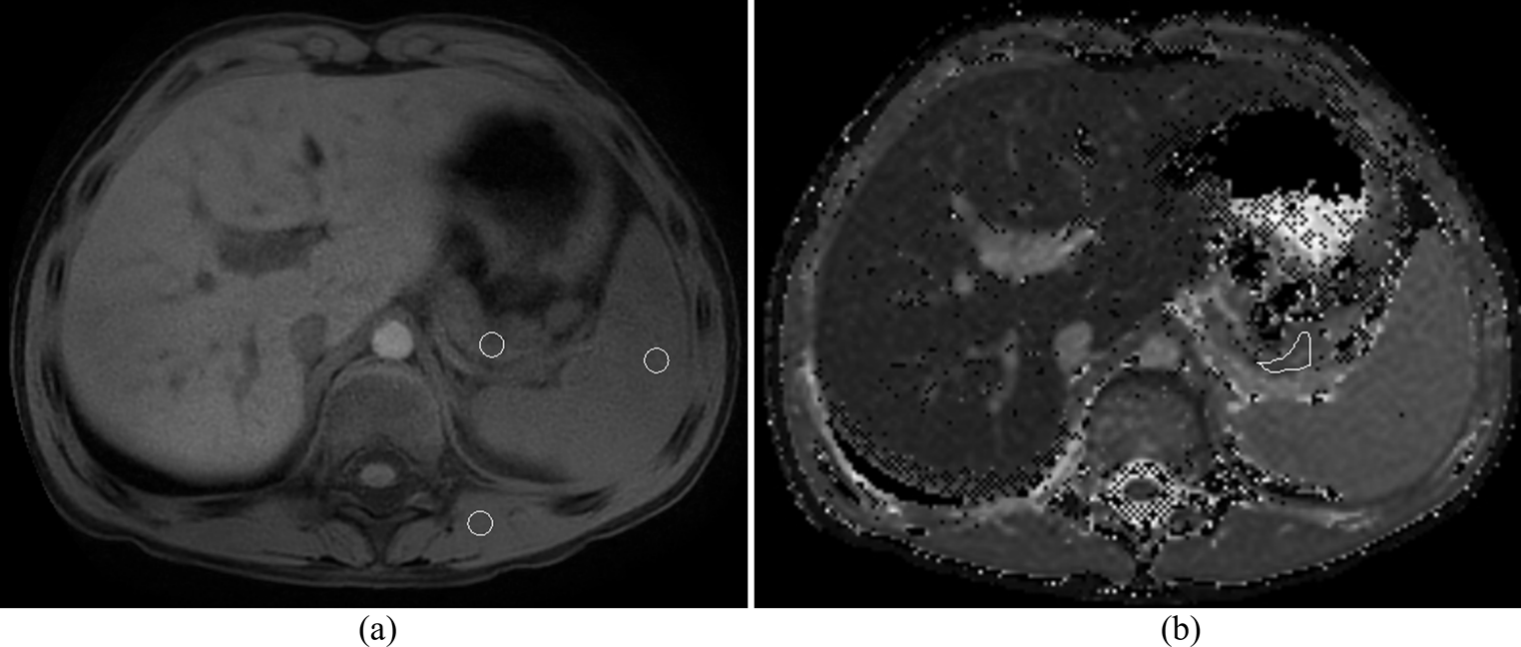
Axial 1.5T (A) T1-weighted 3D VANE and (B) T1 parametric map images in a 7-year-old boy with chronic pancreatitis. Circular regions of interest (ROIs) in the pancreas, spleen, and paraspinal muscle on T1-weighted 3D VANE images were used to calculate T1 weighted signal intensity ratios (T1 SIR). An irregular ROI drawn on the pancreas on the corresponding slice on the T1 parametric map was used to measure estimated T1 relaxation time. ROIs are indicated using a white outline. Pancreas:spleen T1 SIR was 1.02 and Pancreas:paraspinal muscle T1 SIR was 0.93 with corresponding estimated T1 relaxation time of 1053 msec
